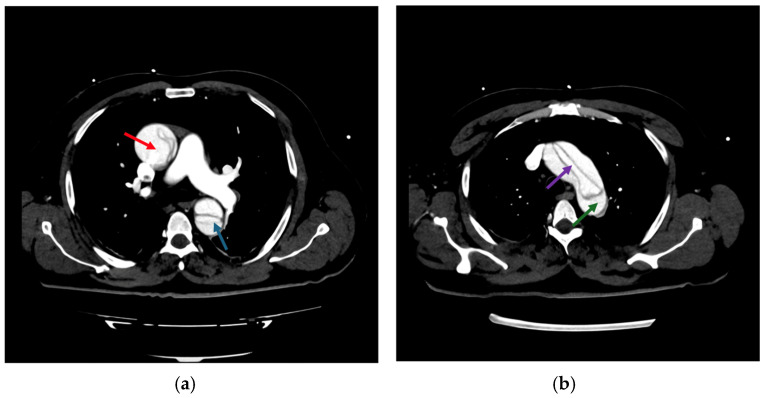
CT imaging of Stanford Type A aortic dissection: true and false lumen analysis—transverse sections: (a) compression of the true lumen (purple arrow) by the false lumen (green arrow) within the ascending aorta indicates compromised blood flow, necessitating immediate surgical intervention to restore adequate perfusion; (b) involvement of the descending thoracic aorta shows significant expansion of the false lumen (red arrow), with partial thrombosis and dynamic obstruction impacting distal perfusion (blue arrow). These findings underscored the urgency of surgical repair to prevent ischemic complications.
